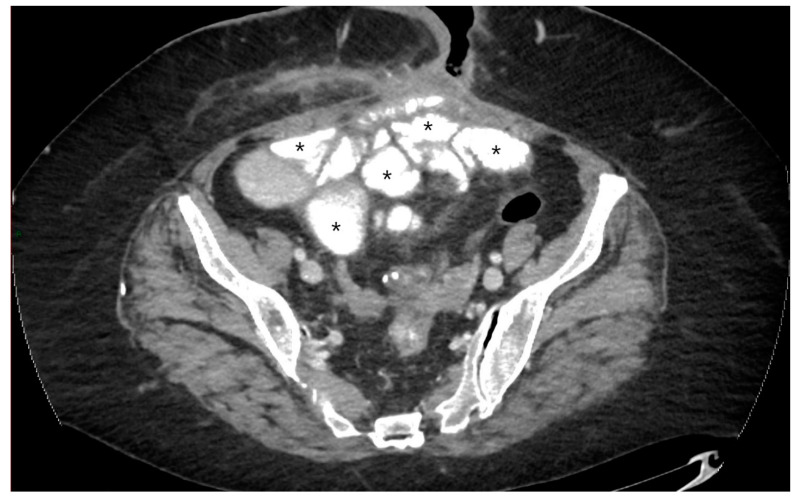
CT-E axial image: example of intestinal distension by oral administration of hydrosoluble iodine contrast medium in patient who has undergone intestinal resection with suspected post-surgical fistula. The image shows the hyperdense appearance of the ileal lumen (asterisks). Original figures by LMM and LL.
